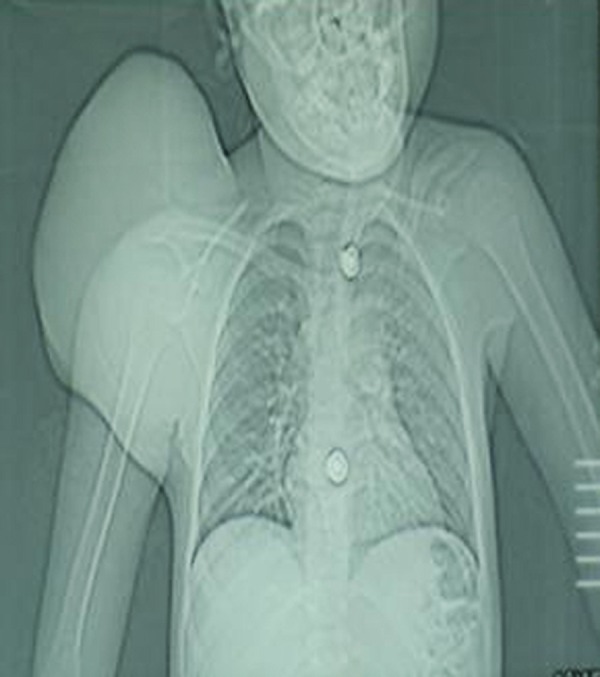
Figure 2: CT scout view showing soft tissue shadow
